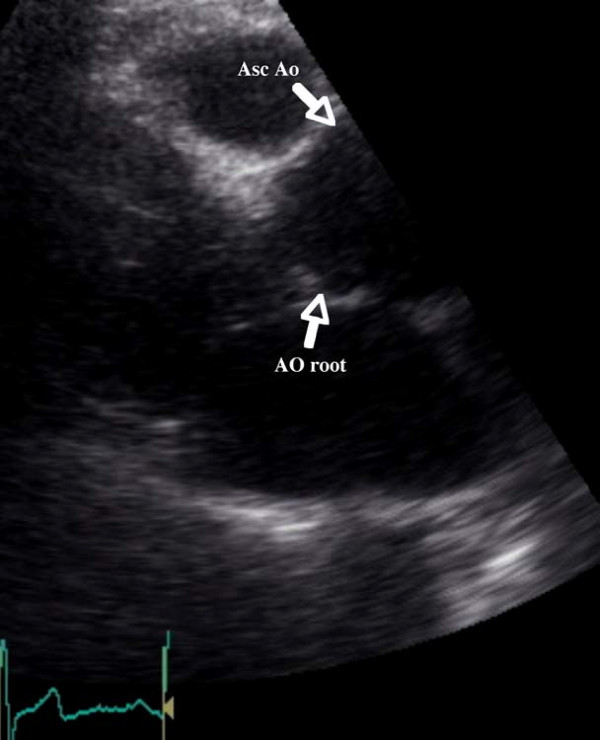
Parasternal long-axis view showing a dilated aortic root and ascending aorta. Ao: Aorta; Asc Ao: Ascending aorta.
